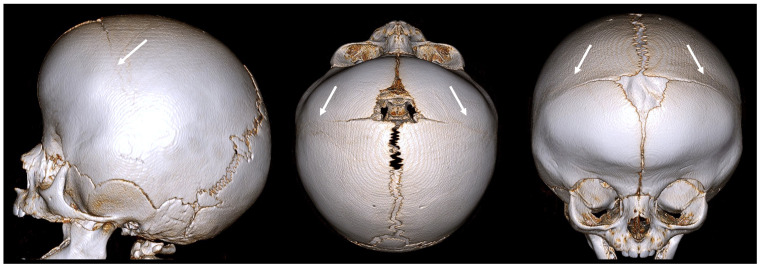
A 3D CT example of bilateral coronal CRS (brachycephaly) with symmetrical flattening of the forehead, short broad skull, and increased ICP due to restricted growth. The white arrow indicates an abnormally fused suture.
